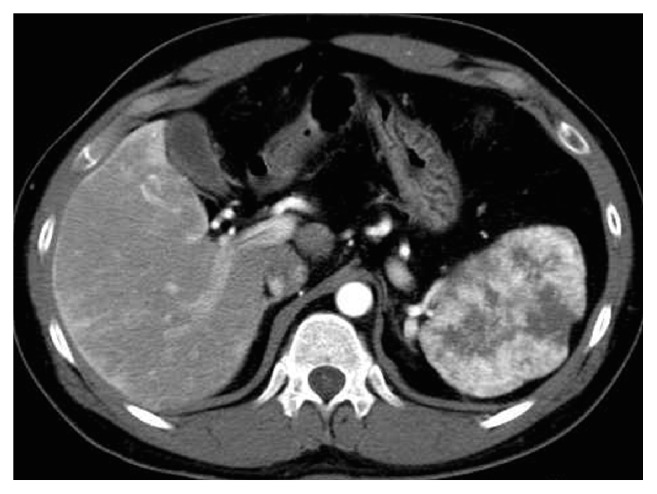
Arterial phase of enhanced CT, showing a 9 cm hypervascular mass in the enlarged spleen of the patient.
